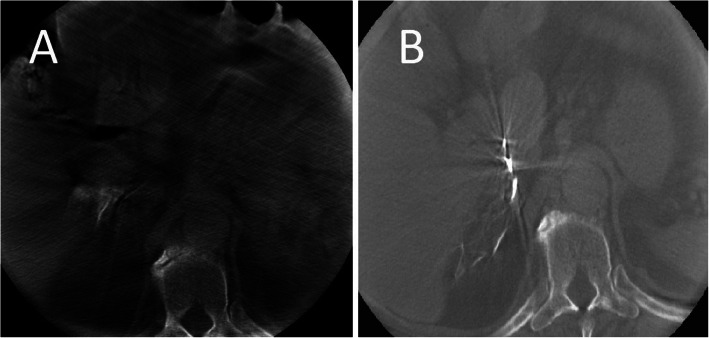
Cone-beam CT of an accessory hepatic vein (a) and the right adrenal vein (b). In this patient, two different veins were cannulated. Given ambiguous conventional venographic appearance, cone-beam CT was performed. a Shows opacification of hepatic parenchyma. b Confirms cannulation of the adrenal vein
